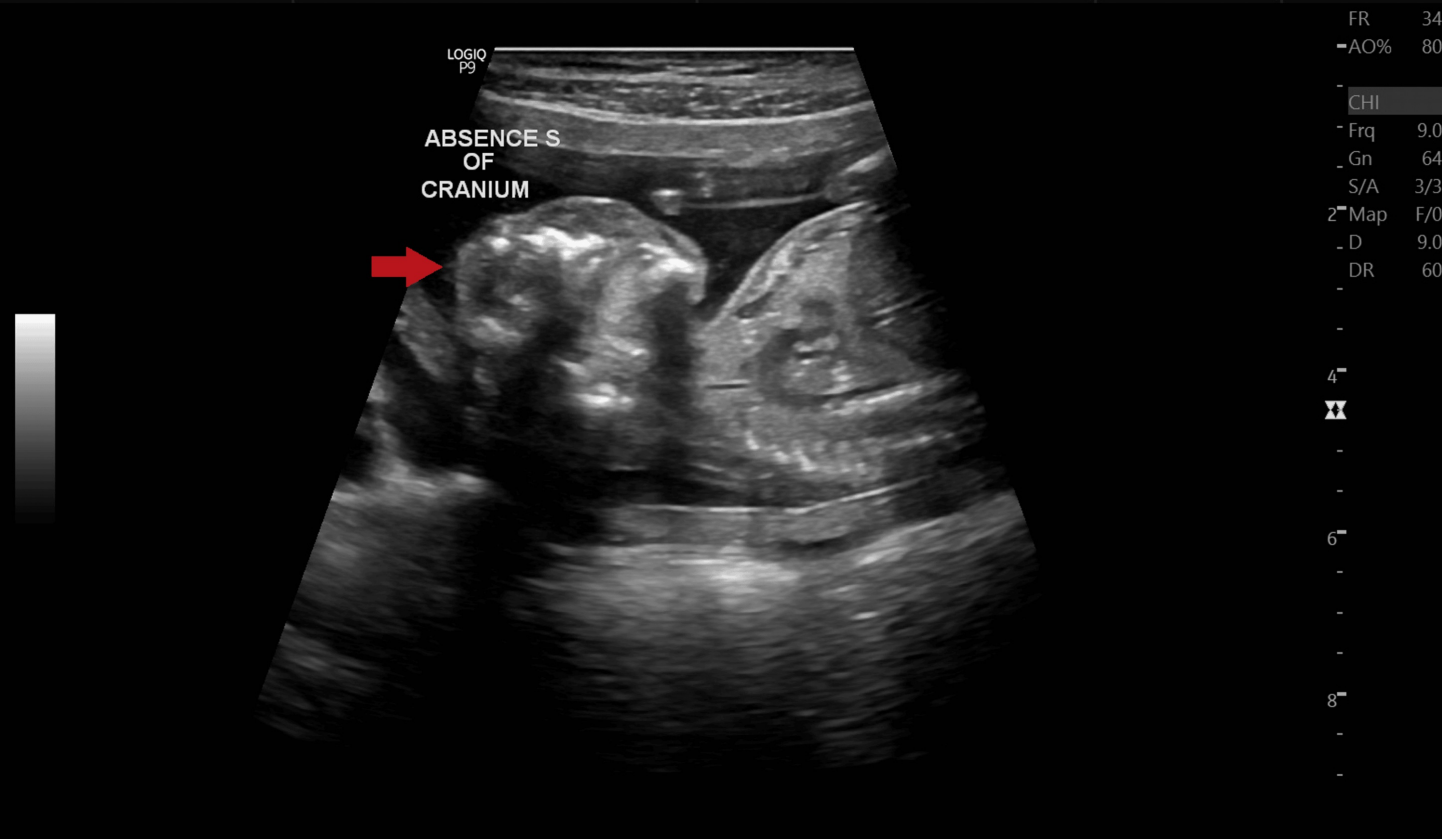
B-mode ultrasonography mid-sagittal image showing the absence of the cranium of the fetus. The arrow (➡) points to the fetal head showing the absence of the cranium.
